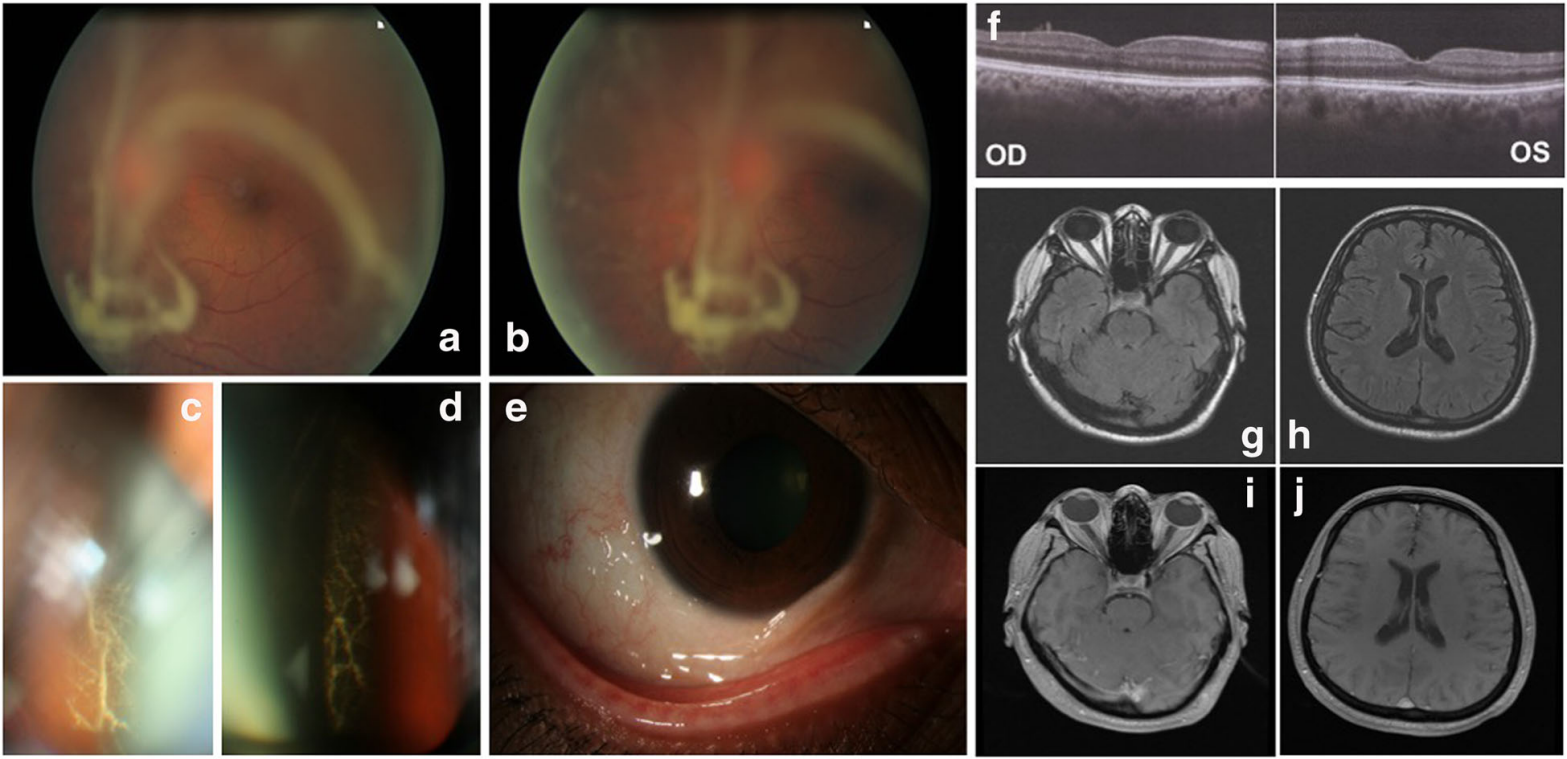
Vitreous amyloidosis. An ocular examination revealed accumulation of extensive fibrillogranular deposits in the vitreous consistent with amyloid deposits (panels a, b, c and d), and conjunctivochalasis (panel e). Optical coherence tomography showed normal retinal appearance with a normal macular and foveal thickness (panel f). Magnetic resonance imaging of the brain revealed no leptomeningeal involvement associated with vitreous amyloidosis on T2-weighted fluid-attenuated inversion recovery sequences (panels g and h) and gadolinium-enhanced T1-weighted sequences (panels i and j). *OD* right eye, *OS* left eye
